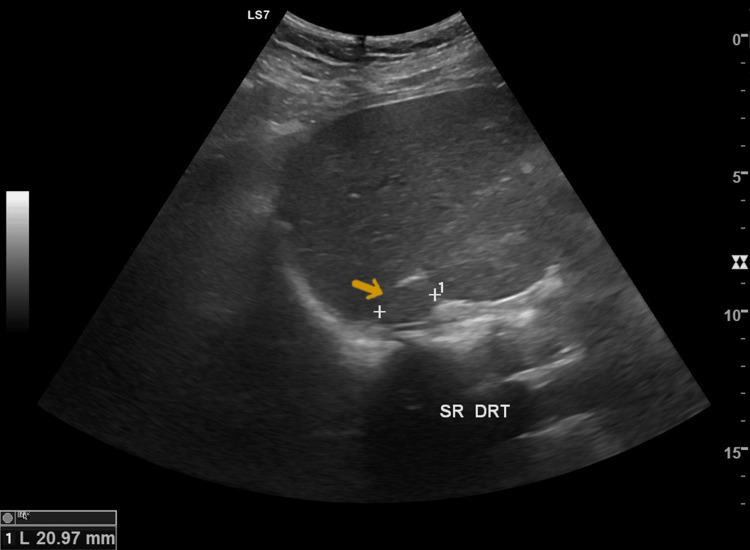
Abdominal ultrasonography revealing a 21-mm nodule in the right adrenal gland (arrow)
